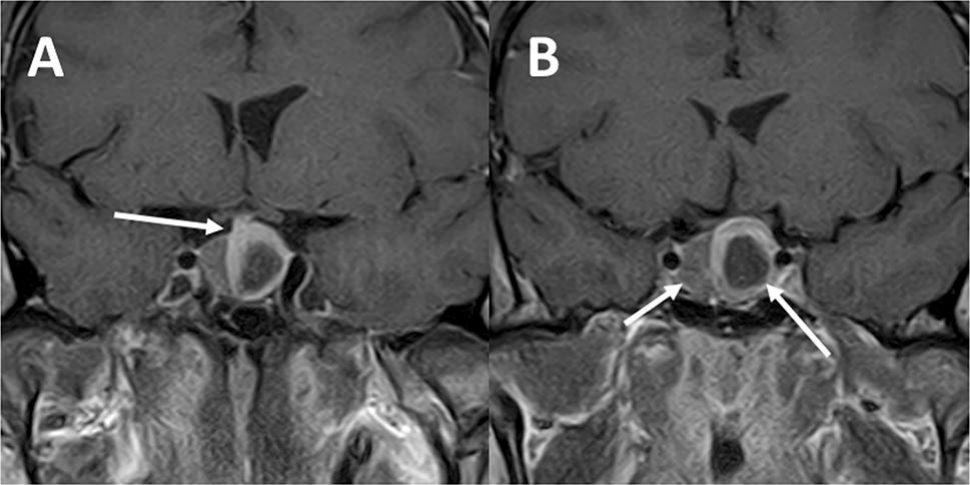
Case no. 9: a coronal T1 weighted MRI after Gd-DTPA revealed two clearly separate pituitary adenomas, b left arrow indicates intrasellar gonadotroph pituitary macroadenoma and right arrow shows cystic somatotroph macroadenoma with suprasellar extension
